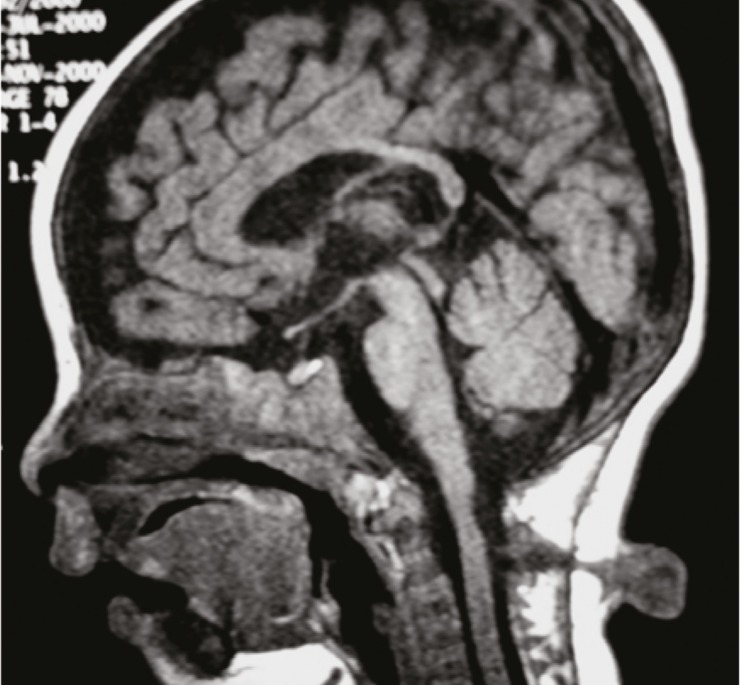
Cervical sagittal T1 weighted MRI shows cervical myelomeningocele at the C2-C3 level
